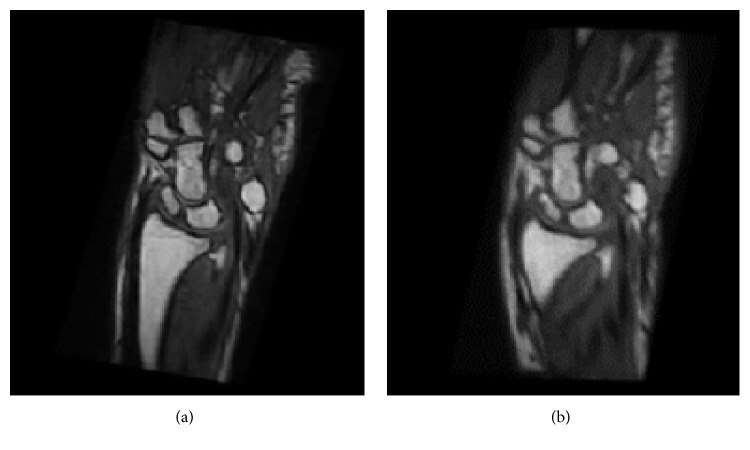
Corresponding sample slices from the first initial MRI sequence (a) and the follow-up sequence (b) after registration of the capitate bone.
